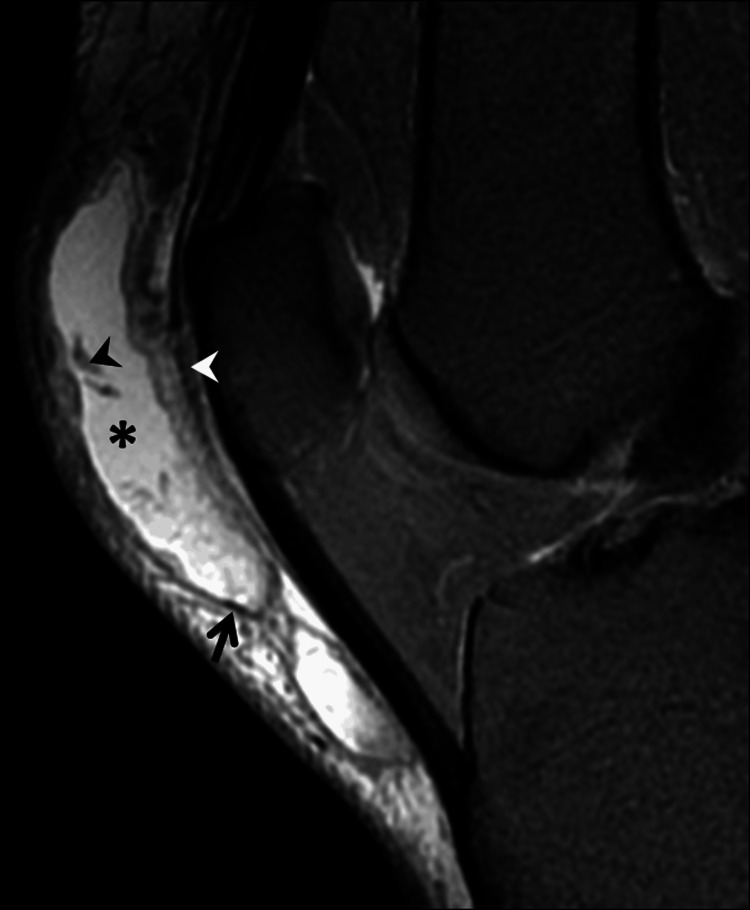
Sagittal view of PD-FS MRI sequence demonstrating a hyperintense prepatellar fluid collection (asterisk) containing hemorrhagic debris (black arrowhead) and featuring a well-defined capsule (black arrow). Note the longitudinal fibers of the rectus femoris tendon extending to the patellar tendon (white arrowhead). PD-FS, proton density fat-saturated; MRI, magnetic resonance imaging
